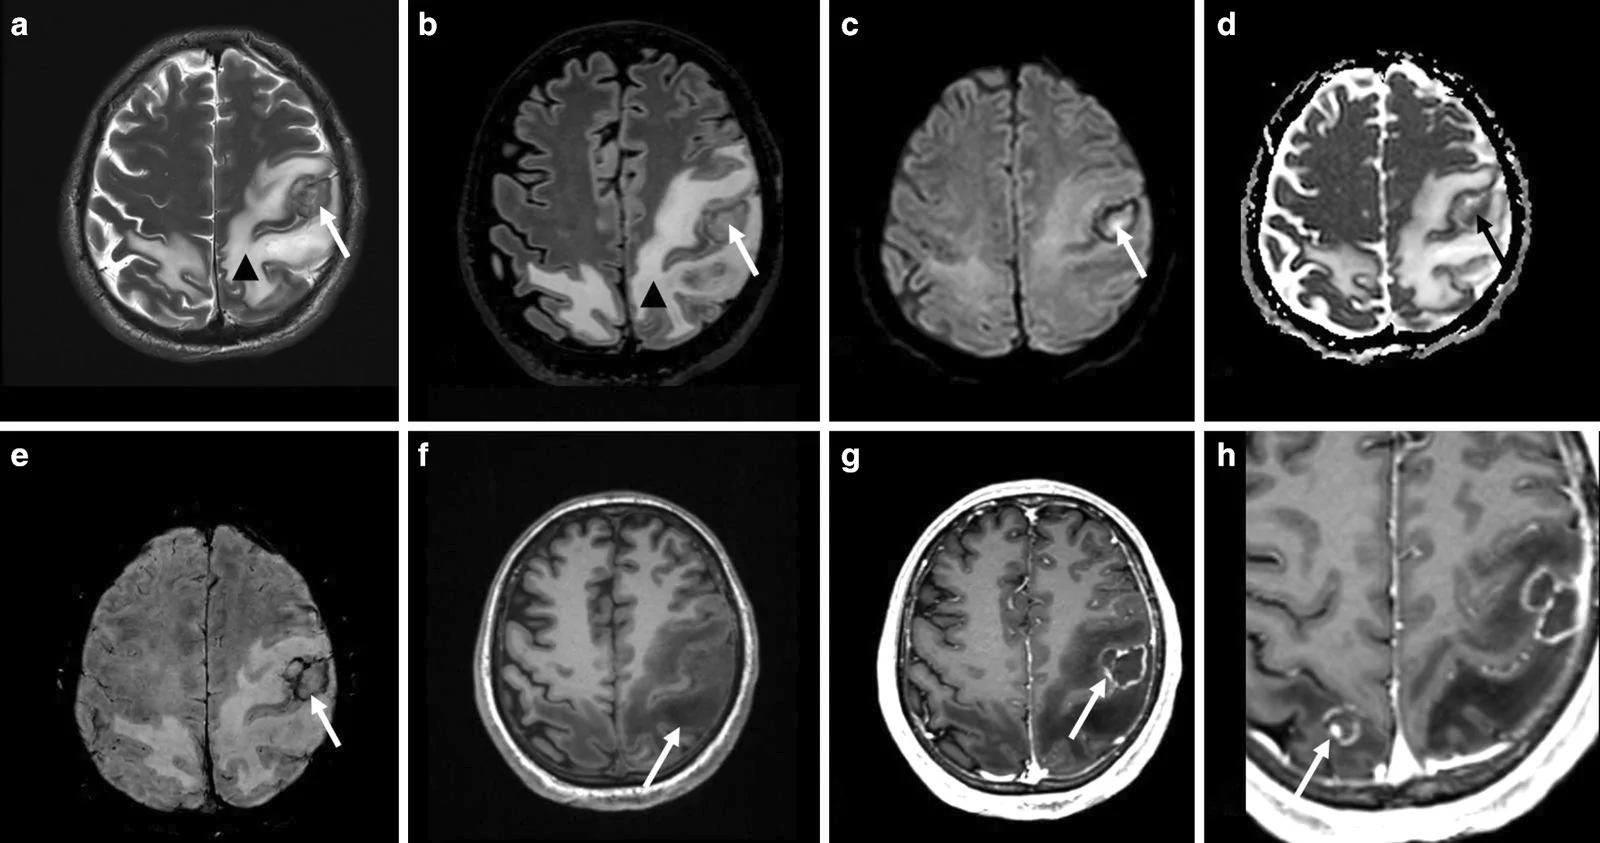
Toxoplasmosis. 39-year old man with HIV infection suffering from right-sided progressive hemiparesis and recurrent epileptic seizures. a–g inhomogeneous hyperintense lesion in the left-sided central region on T2 weighted images (WI) (a, b arrow; b fluid attenuated inversion recovery (FLAIR)) with extensive perifocal edema (a, b black arrowhead), restricted diffusion (c, d arrow; c DWI; d apparent diffusion coefficient (ADC) map; ADC-value: 0.703 ± 0.042 × 10^−3^ mm^2^/s; mean ± SD; d arrow); marginal signal loss on susceptibility WI (SWI) (e arrow) and inhomogeneous contrast enhancement (f, g arrow; f T1 WI; g, h post contrast T1 WI); note excentric target sign in another right-sided postcentral/parietal lesion (h arrow)
